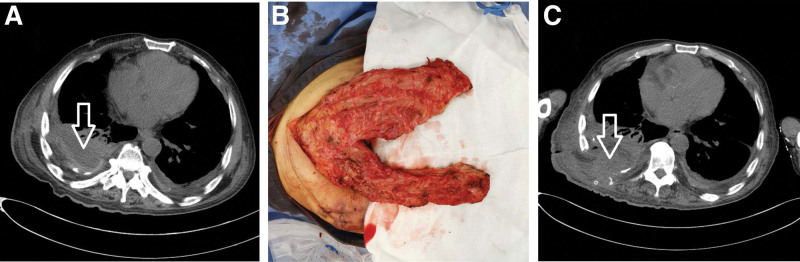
Patient B had an 80 mL abscess cavity on the dorsal side of the right lung (arrow in Figure a), and the ipsilateral latissimus dorsi muscle was selected to fill the abscess cavity (Figure b). Chest CT was reviewed 19 days after the operation and showed that the abscess cavity had completely disappeared (Figure c).
